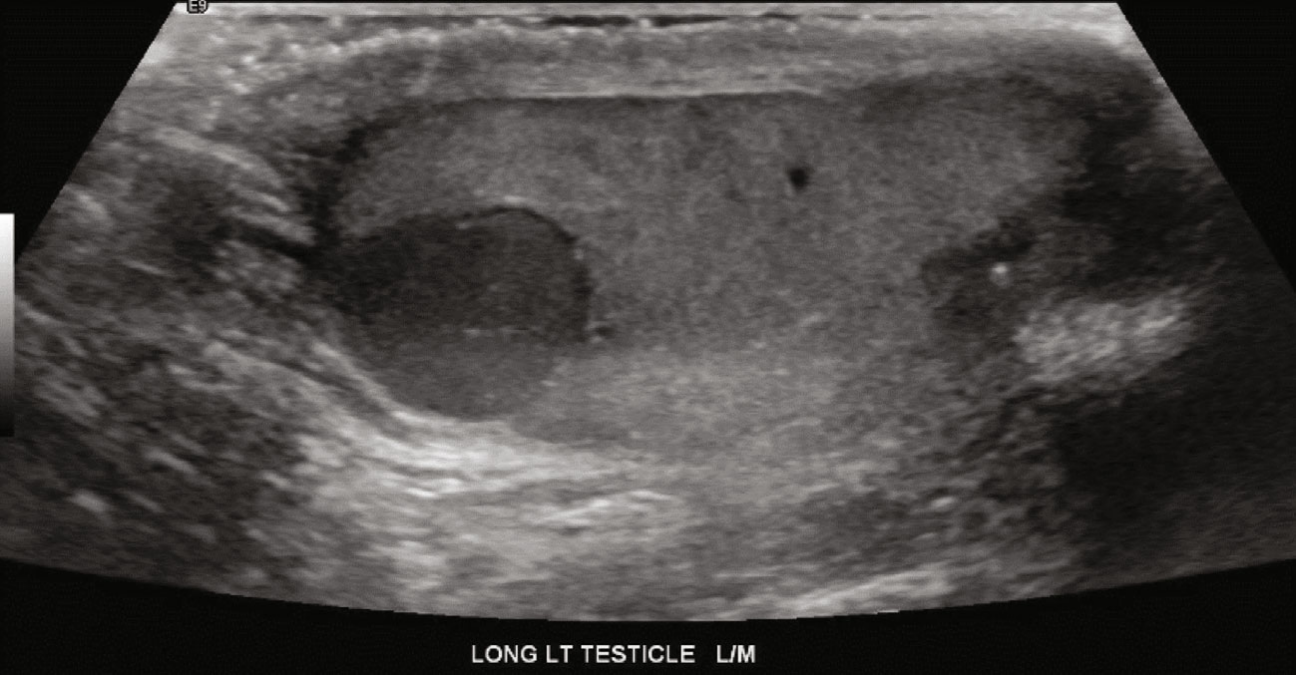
Scrotal ultrasound demonstrating left testis mass.
